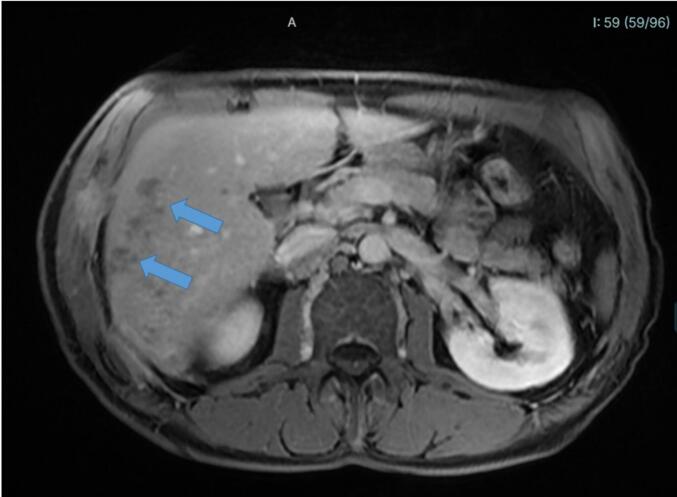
Liver Abdominal Magnetic Resonance Imaging (Lava-Flex T1 sequence) in a patient diagnosed with fasciolosis. The figure is notable for the presence of tunnel-like lesions (arrows), which are typically associated with hepatic fasciolosis.
